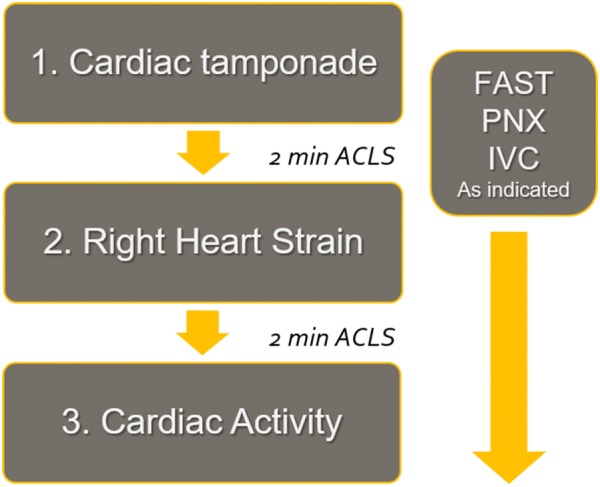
The Cardiac Arrest Sonographic Assessment (CASA) protocol. Reproduced with author’s permission from the original algorithm curtesy of Gardner et al. [26]
